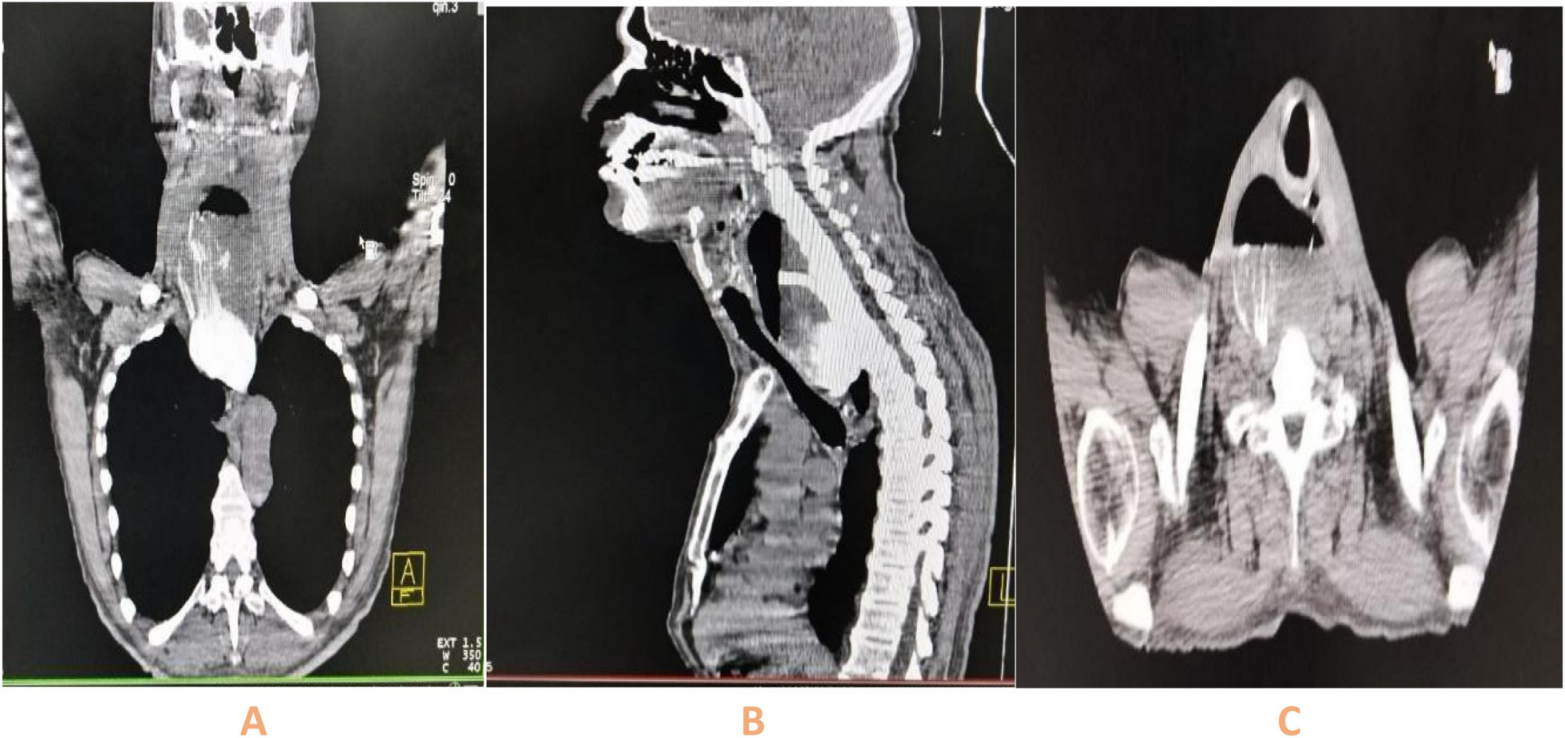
Coronal (A), sagittal (B), and axial (C) CT scans with Gastrografin contrast performed 10 days after open diverticulectomy demonstrate a large air-fluid collection adjacent to Killian's dehiscence, measuring 14 × 7.2 × 5.5 cm, lateralized to the right of the esophagus and extending inferiorly to the upper sternum. Extravasation of contrast from the hypopharynx into the collection confirms communication and establishes the diagnosis of a hypopharyngeal fistula.
